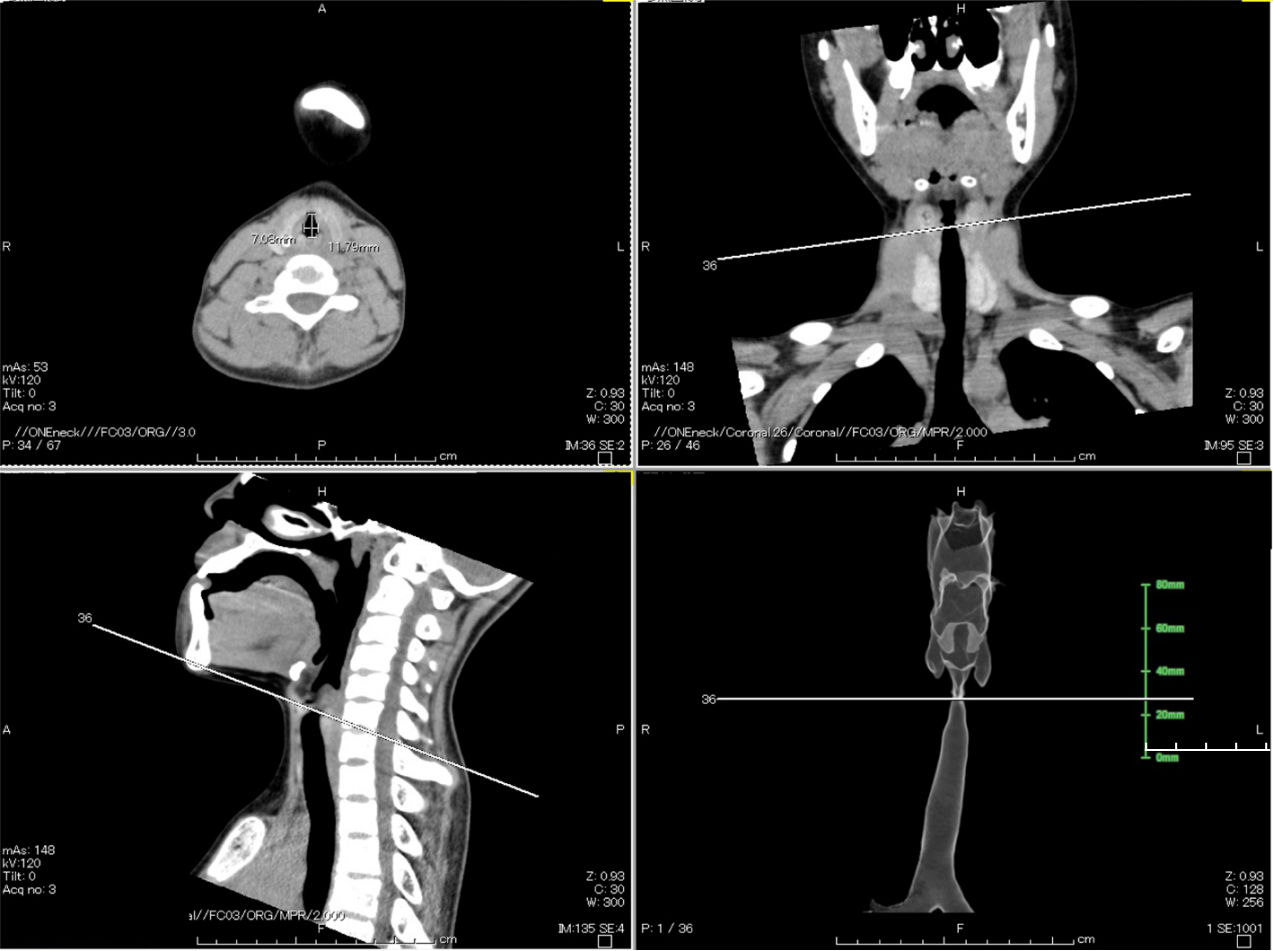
Cervical computed tomography showed the narrowest site of the airway was in laryngeal region, not at the lower airway levels
